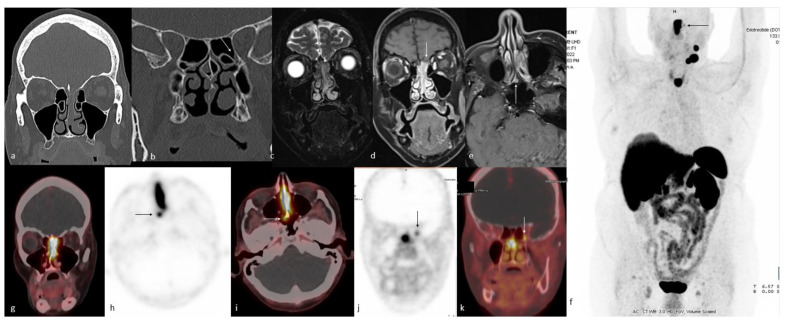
Olfactory neuroblastoma with metastases. A 71-year-old female presenting with a palpable left neck mass. CT (a,b) demonstrated a left nasal cavity mass with dehiscence of the cribriform plate on the left side. MRI images [coronal fat-saturated T2w (c), post-contrast fat-saturated T1w (d,e)] demonstrate a T2w isointense, enhancing left nasal cavity mass invading the olfactory fossa (arrow, (d)). The biopsy of the left nasal cavity mass was consistent with grade 2 olfactory neuroblastoma. Subsequently, ^68^Ga-DOTATOC PET/CT was performed. The MIP image (f) shows intense uptake in the left nasal cavity mass, with a small focus of uptake just laterally (arrow, (f)), and uptake in the left level IB and IIB lymph nodes and thyroid gland. Fused coronal (g) and axial (i) images show intense FDG uptake in the nasal cavity lesion with a small right paramidline focus of uptake at the level of the choanae (arrow (h,i)). Retrospectively, this can be identified as an enhancing focus on MRI (arrow, (e)). There is also another focus of uptake near the left orbital apex in the vicinity of the left posterior ethmoid air cells (arrow, (j,k)), which can be identified retrospectively as an area of mucosal thickening on initial CT (arrow, (b)).
